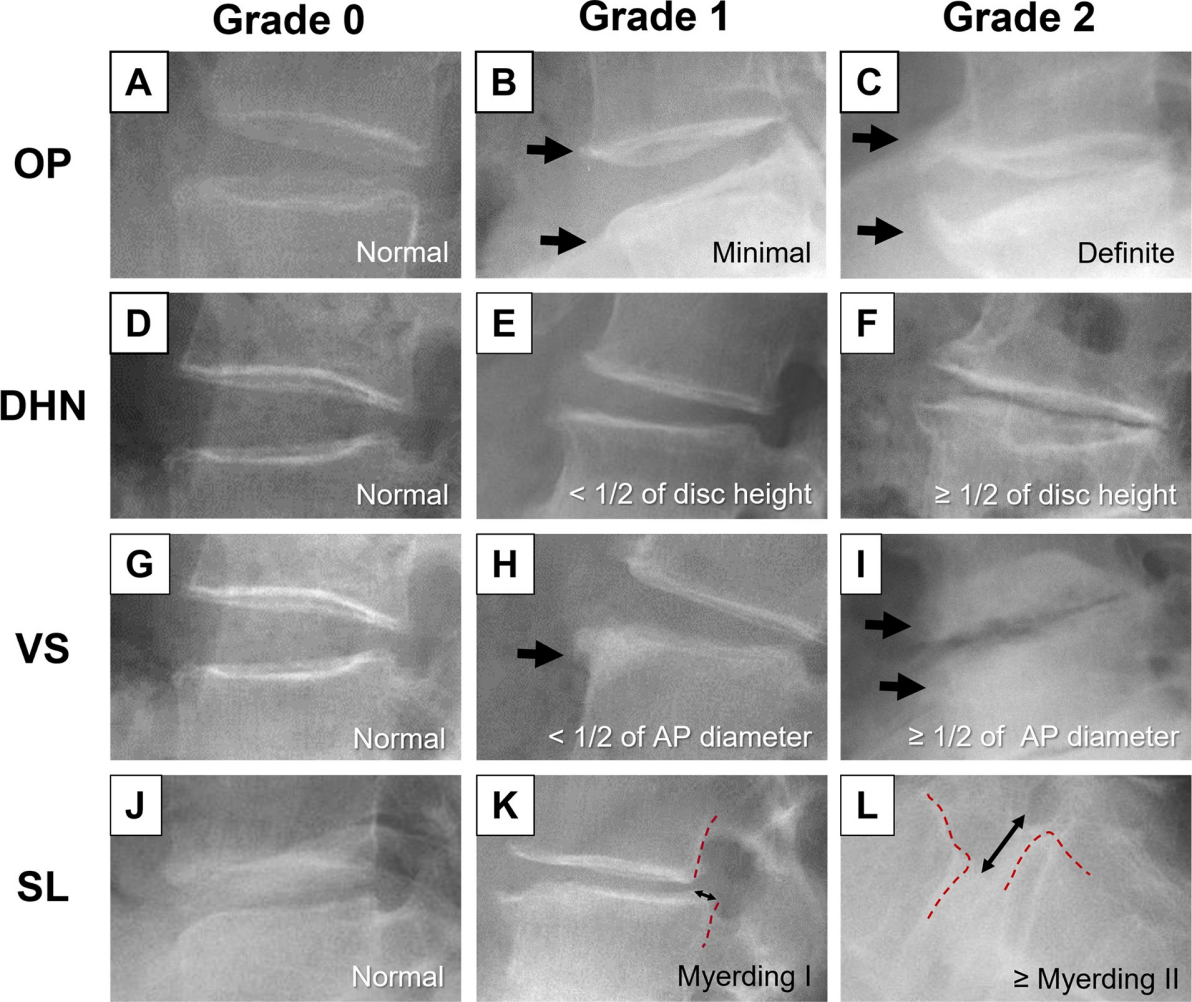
Typical radiographic features for the novel elemental grading system. Osteophytes (OP) were classified as grade 0, normal (A); grade 1, minimal anterior vertebral osteophytosis (B); and grade 2, definite vertebral osteophytosis (C). Disc height narrowing (DHN) was classified as grade 0, normal (D); grade 1, mild (less than 1/2 of estimated disc height) DHN (E); and grade 2, severe (equal to or more than 1/2 of estimated disc height) DHN (F). Vertebral sclerosis (VS) was classified into grade 0, normal (G); grade 1, mild (less than 1/2 of anteroposterior diameter) sclerosis (H); and grade 2, severe (equal to or more than 1/2 of anteroposterior diameter) sclerosis (I). Spondylolisthesis (SL) was classified into grade 0, normal (J); grade 1, Meyerding [15] grade I (K); and grade 2, Meyerding grade II or worse (L).
